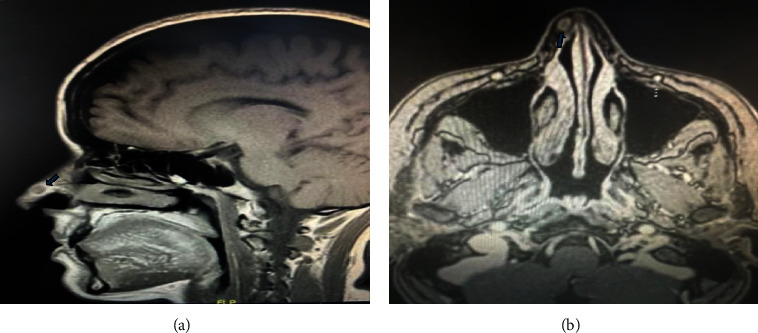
Sagittal (a) and axial (b) magnetic resonance imaging confirmed the presence of an oval lesion (arrows) measuring about 0.5 x 0.7 cm with isointense signals in all pulse sequences, with homogenous postcontrast enhancement, clear surrounding fat planes with no invasion to nasal septum or other adjacent structures.
